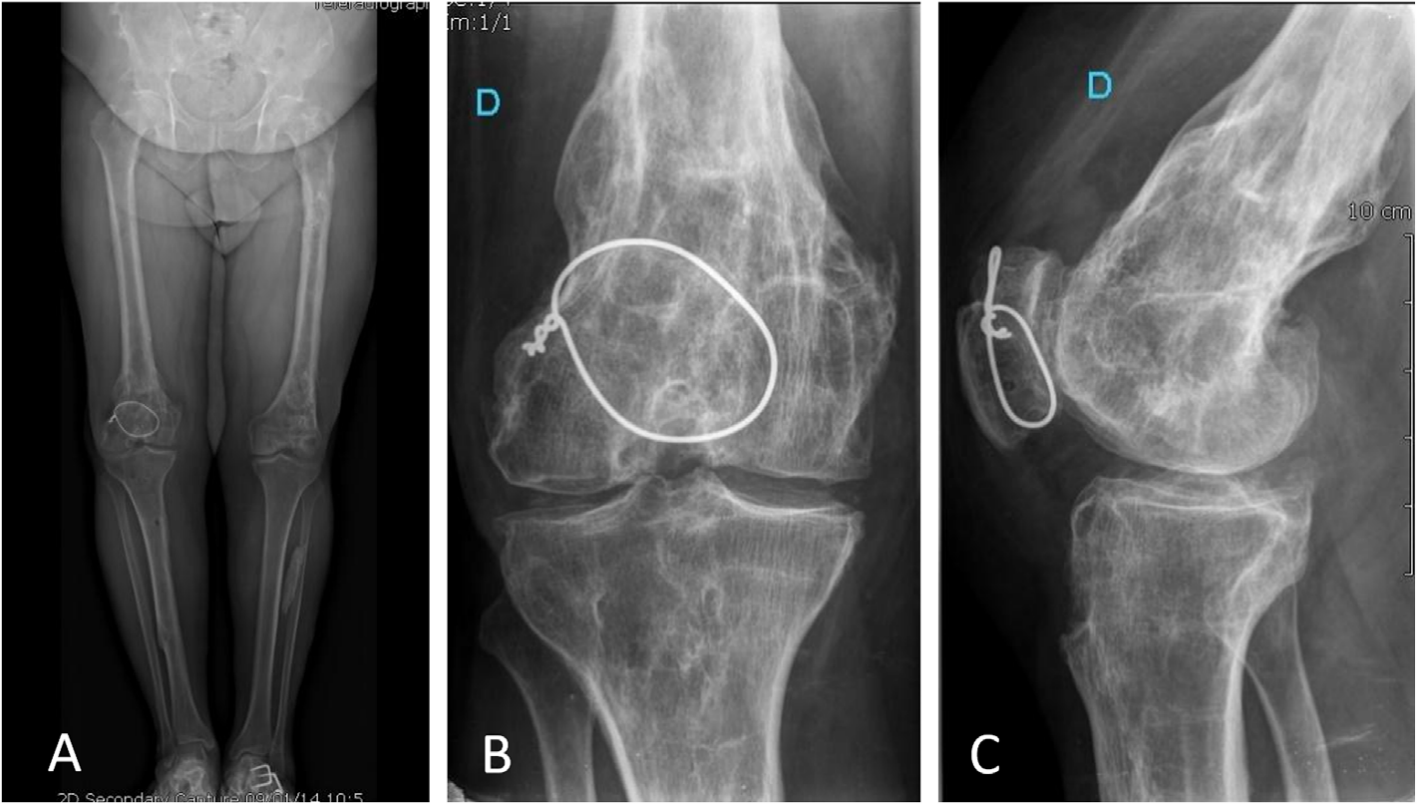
Pre-operative plain radiographs: (A) standing bilateral, (B) AP view, right knee, (C) lateral view, right knee.
